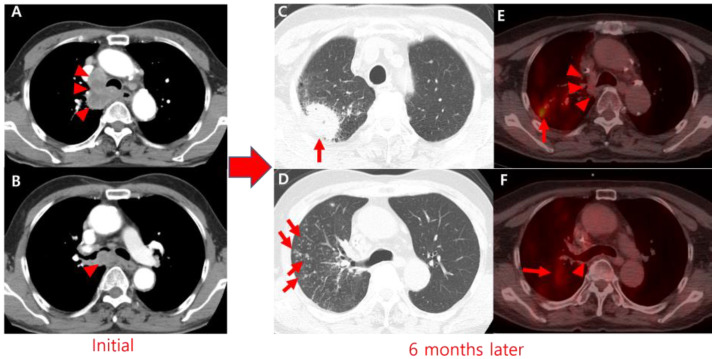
(A,B) A 67-year-old man with small-cell lung cancer (arrowheads) who underwent chemotherapy with etoposide and cisplatin. (C,D) After 6 months, he developed a cough and sputum production. Chest computed tomography (CT) (120 kVp; automatic exposure control; slice thickness, 3 mm) and 2-deoxy-2-[^18^F] fluoro-D-glucose (FDG) positron emission tomography/computed tomography (PET/CT) (Discovery STe, General Electric Healthcare, Milwaukee, WI, USA; 5.5 MBq/kg of FDG; uptake time, 60 min; iterative reconstruction with CT-based attenuation correction) were performed. Chest CT revealed multifocal consolidations and centrilobular nodules in the right upper lobe (RUL, red arrows). Pulmonary tuberculosis (TB) was confirmed by positive sputum cultures for *Mycobacterium tuberculosis*, and antituberculosis therapy was initiated. (E,F) PET/CT demonstrated increased FDG uptake (red arrows) in the newly developed consolidations and nodules, while the small-cell lung cancer had resolved (arrowheads).
